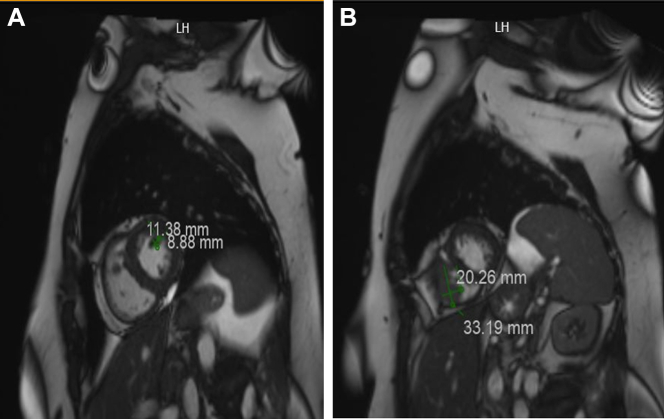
Findings on Cardiac Magnetic Resonance Imaging Cardiac magnetic resonance imaging showing (A) a 3.3-cm irregular mass in the right ventricle and (B) a 1.1-cm oval mass in the left ventricle.
